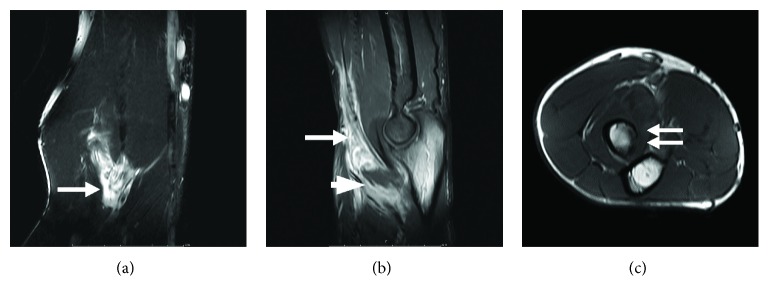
MRI demonstrating a (a) coronal T2-weighted fat-saturated image, (b) sagittal T1-weighted fat-suppressed image with contrast administration, and (c) axial T1-weighted image that showed a disruption of the biceps tendon (arrow in a and b) with ill-defined soft tissue mass (about 2 × 2 cm.). A less enhanced area was noted at the distal part, which was likely to be necrotic or cystic (arrowhead in b). An abnormal marrow signal was detected at the proximal radius with focal cortical erosion at the radial tuberosity (double arrow in c).
